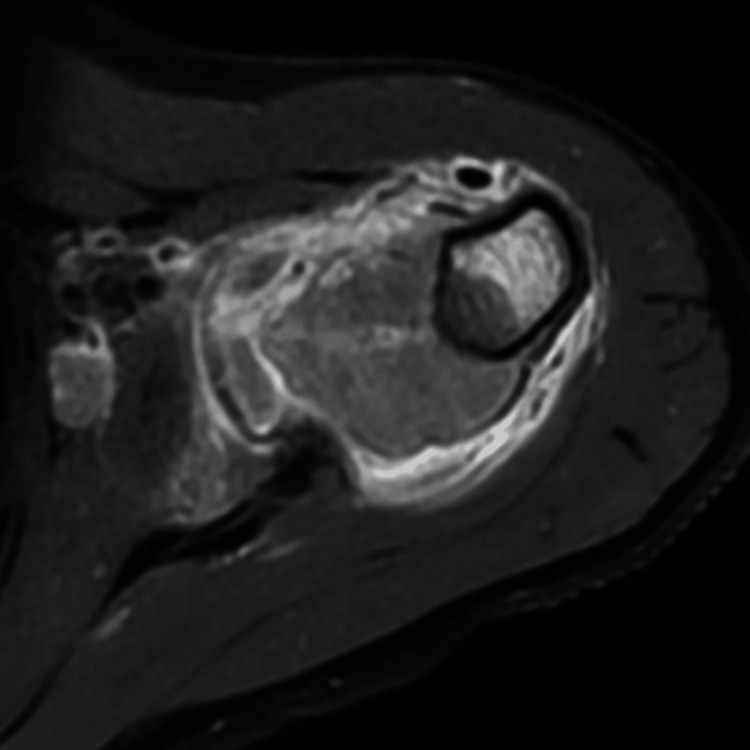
Post-contrast T1-weighted fat-saturated axial image of left shoulder Mildly enhancing mass and enlarged enhancing left axillary lymph node is seen.
